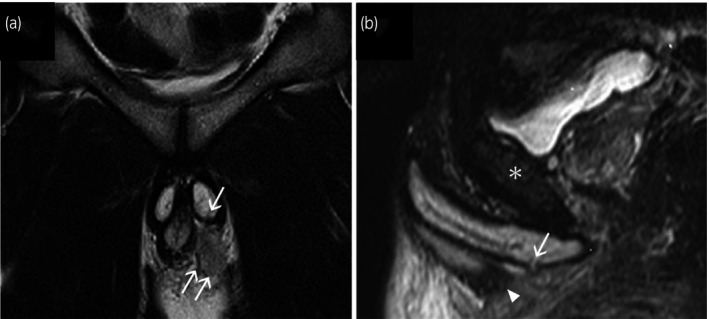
(a) Pelvic MRI coronal T2WI and (b) sagittal T2WI fat suppression. The 6‐mm tear in the tunica albuginea of the left ventral penile crus (single arrow) and a hematoma (double arrows) are presented. Arrowhead points to the bulbospongiosus muscle. The asterisk shows the pubic bone.
